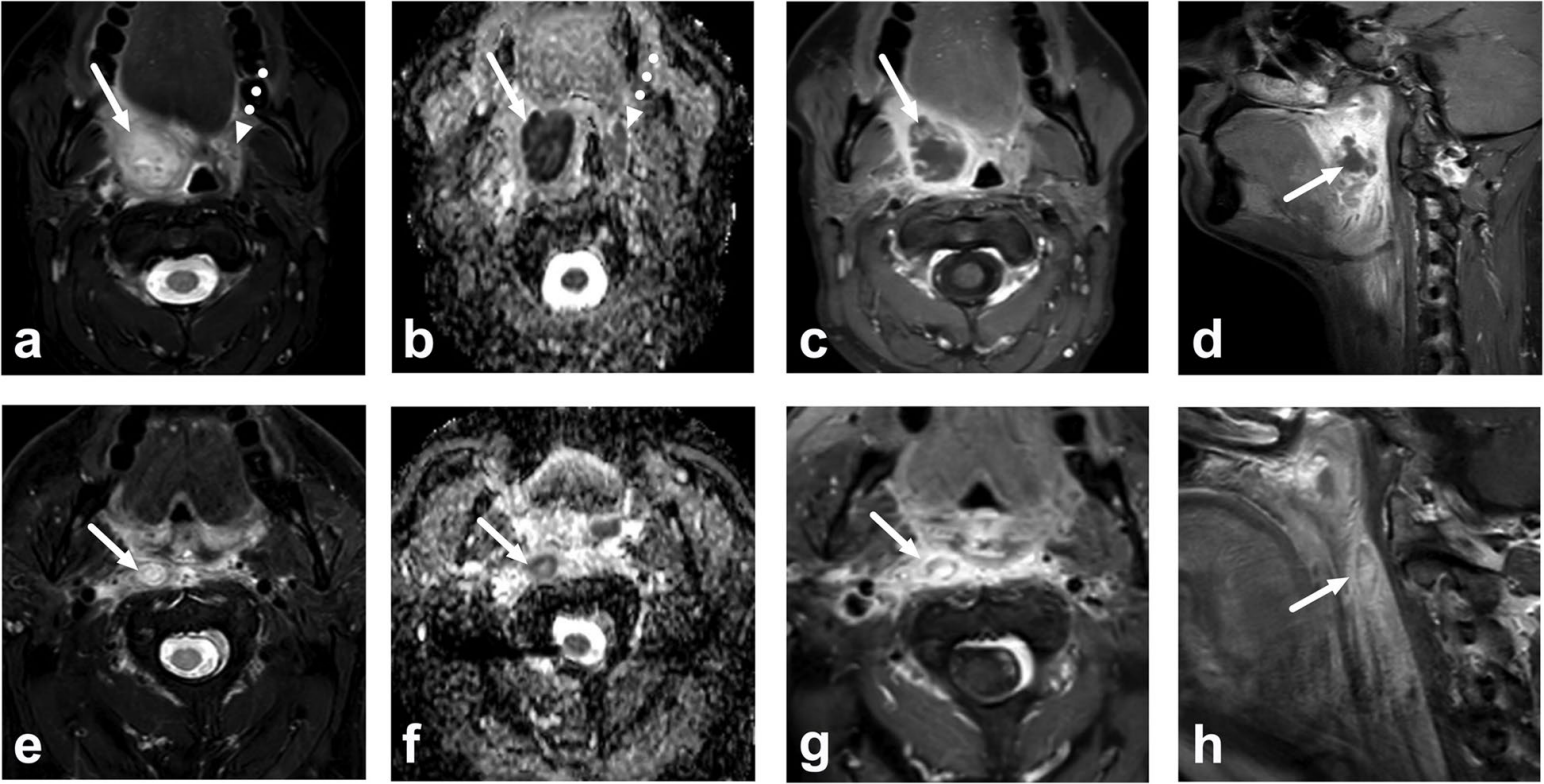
Examples of a true positive (a–d) and a false positive (e–h) peritonsillar abscess (PTA) interpreted using the short protocol. Images are axial fat-suppressed T2-weighted images (a and e), apparent diffusion coefficient (ADC) maps (b and f), and axial (c and g) and sagittal (d and h) postcontrast T1-weighted images. True positive PTA, top row (a–d): a T2-hyperintense collection with low ADC (arrows) can easily be separated from normal tonsillar tissue (dotted arrow). All three radiologists correctly identified the PTA using the short protocol (a and b) and subsequently using contrast-enhanced images (c and d). False positive case, bottom row (e–h): a small T2-hyperintense (e) area with low ADC (f) can be seen in the retropharyngeal space (arrows), interpreted as a PTA by one of three radiologists using the short protocol. However, postcontrast images (g and h) clearly demonstrate contrast enhancement in this ovoid lesion (arrows), thus ruling out an abscess and suggesting a retropharyngeal lymph node
